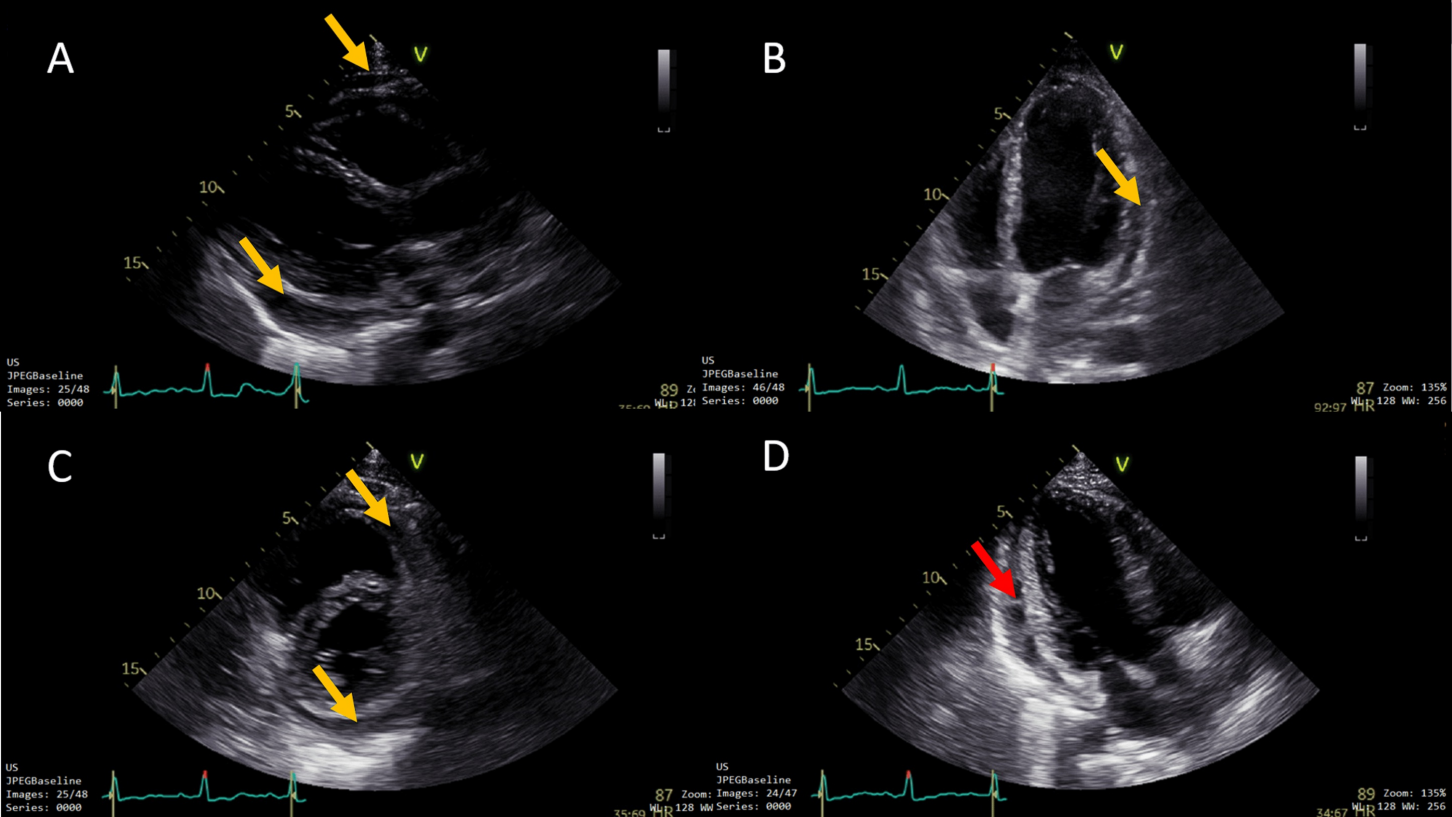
Transthoracic echocardiography (March 12, 2025). Images show evidence of pericardial effusion (yellow arrows) in the parasternal long-axis view (A), apical four-chamber view (B), parasternal short-axis view (C), and fibrin strands in the apical three-chamber view (D) (red arrow).
